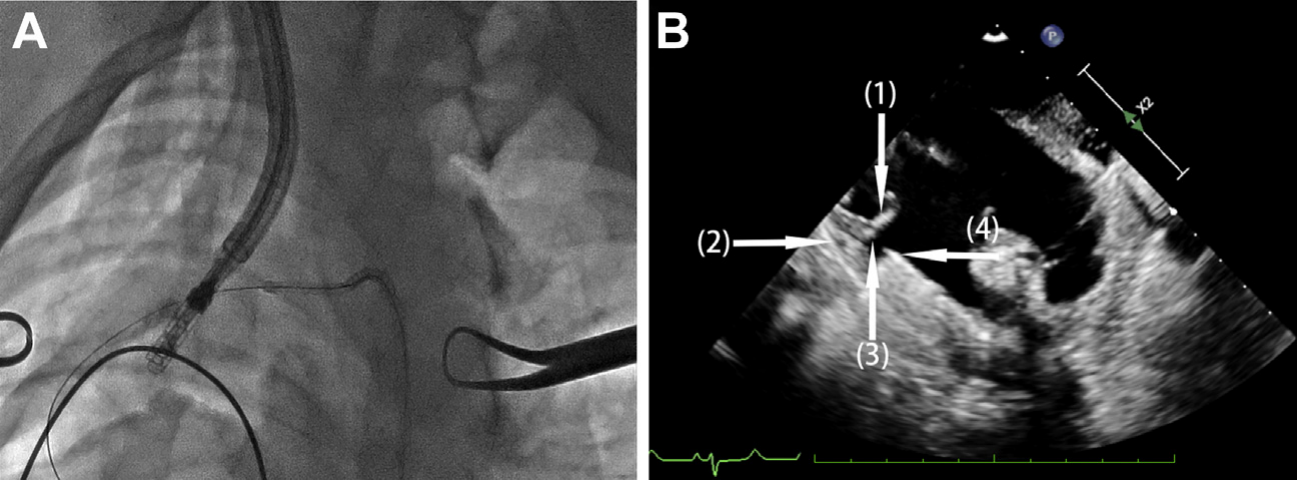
Fluoroscopy and Direct Epicardial Echocardiogram of Annulus Anchoring Procedure (A) The guidewire placed in the right coronary artery in help with identifying the location of the tricuspid annulus on fluoroscopy. (B) In the apical 2-chamber view of echocardiogram, white arrows indicate (1) leaflet issue; (2) right coronary artery; (3) tricuspid annulus; and (4) the distal end of the inner sheath.
